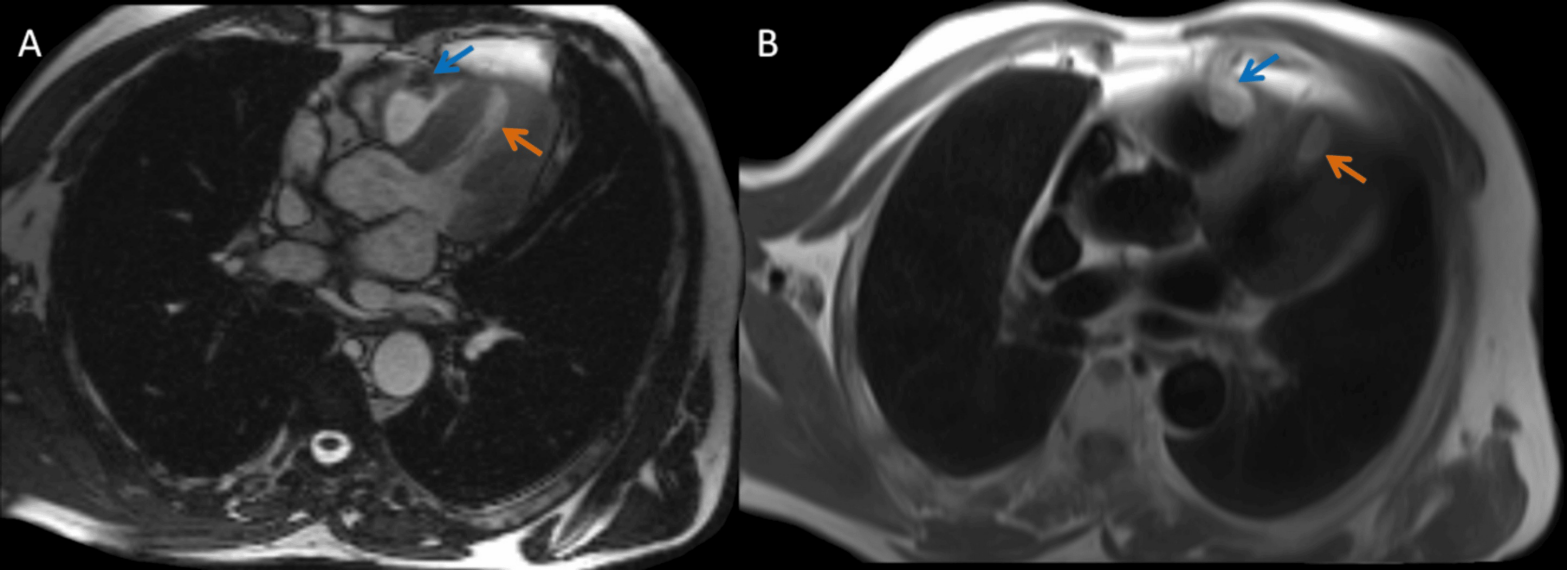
Cardiac MRI sequences: steady-state free precession (SSFP) cine MRI sequence (A) and T1-weighted black blood MRI sequence (B). (A) A bright blood signal. A well-defined, heterogeneous mass is observed in the anterior aspect of the right ventricle (blue arrow). A second lesion is noted within the cardiac apex of the left ventricle presenting with a bright hyperintense signal similar to the surrounding fat (orange arrow). (B) The right (blue arrow) and left (orange arrow) ventricular masses remain visible and exhibit a bright hyperintense signal similar to the surrounding fat. The black blood technique suppresses blood flow, improves visualization of the myocardium and mass effect, and well-defined lesion borders.
